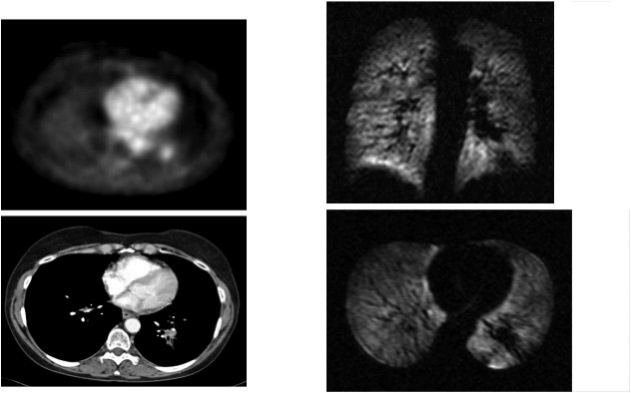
Patient #5 – Small left lingular mass seen on CT, PET, HeMRI.
